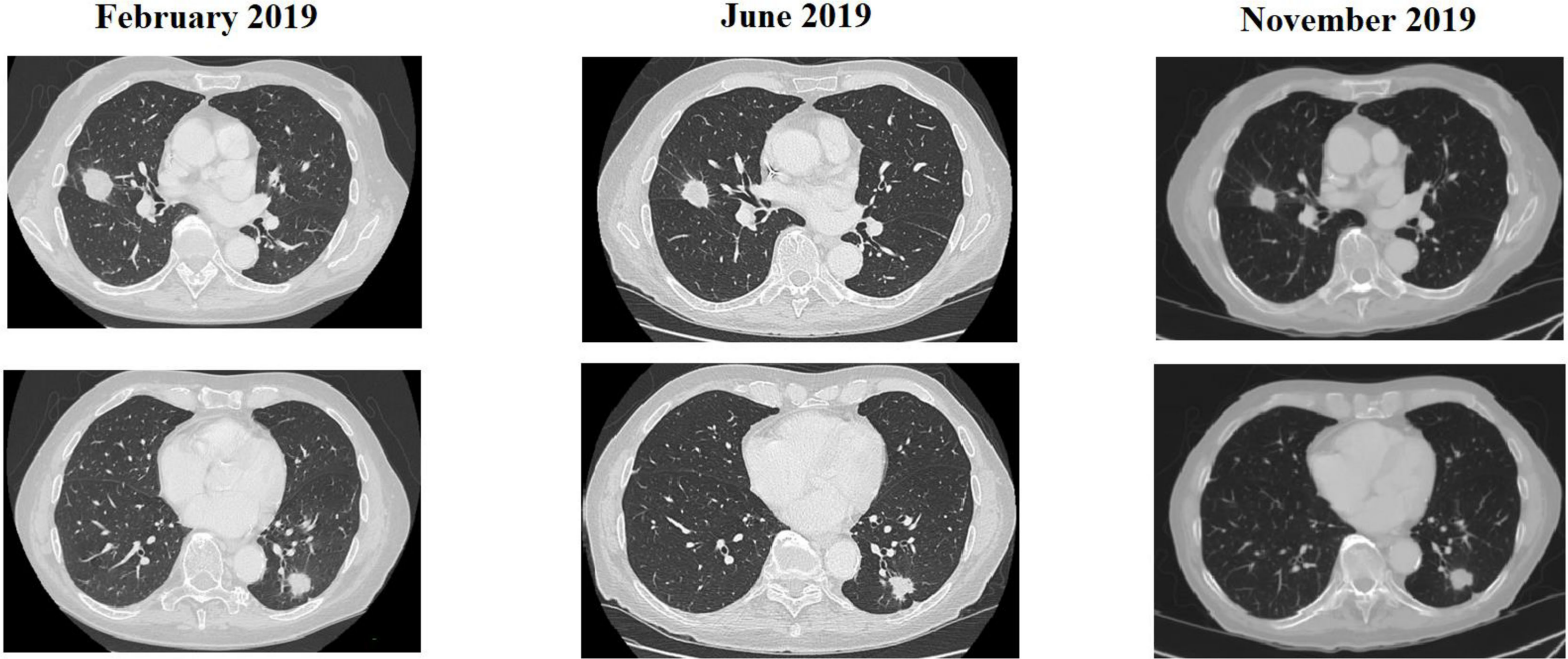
CT scan of a patient treated with rechallenge strategy after >2 prior lines of treatment and an anti-EGFR free interval > 16 months.
